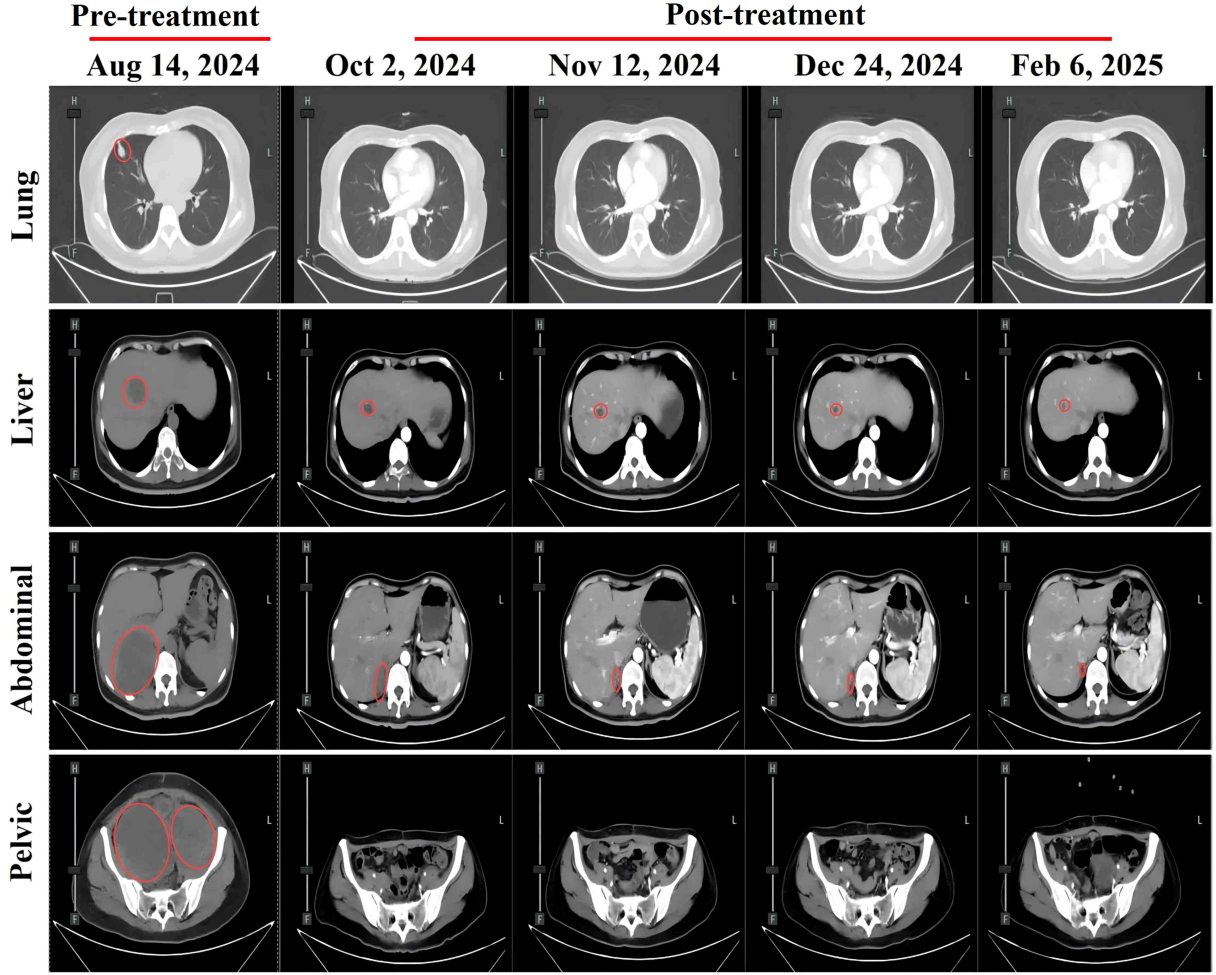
Abdominal CT scans at different treatment time points showing changes in metastatic lesions: pulmonary metastasis, hepatic metastasis, abdominal metastasis, and pelvic metastasis. Red circles indicate metastatic lesions.
